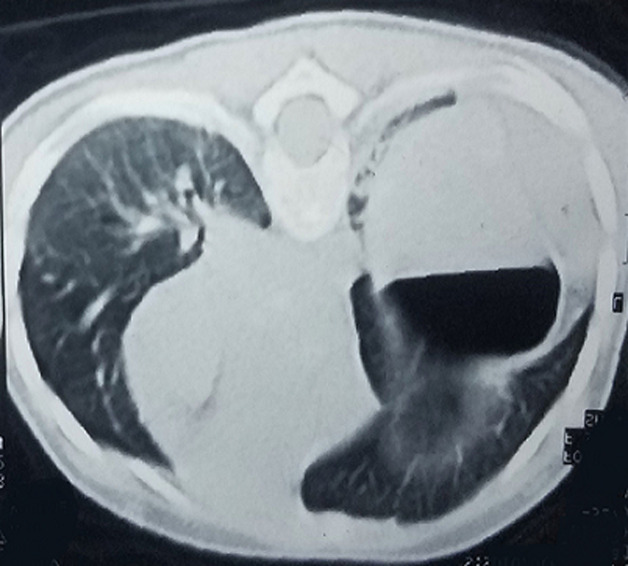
a chest computed tomography scan reveals a large diaphragmatic defect in the left side and complete collapse of the left lung
